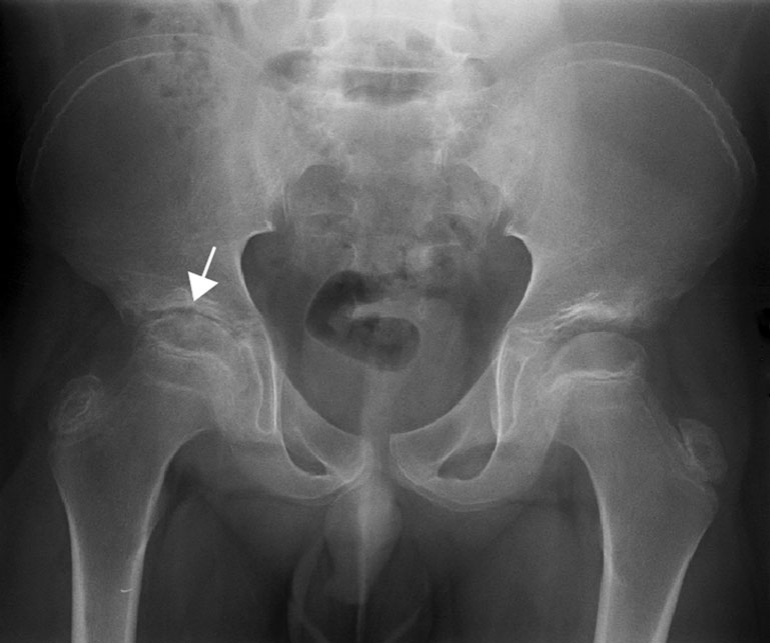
Erosions and joint space narrowing can be seen on radiographs (arrow) but are normally a late finding in arthritis
